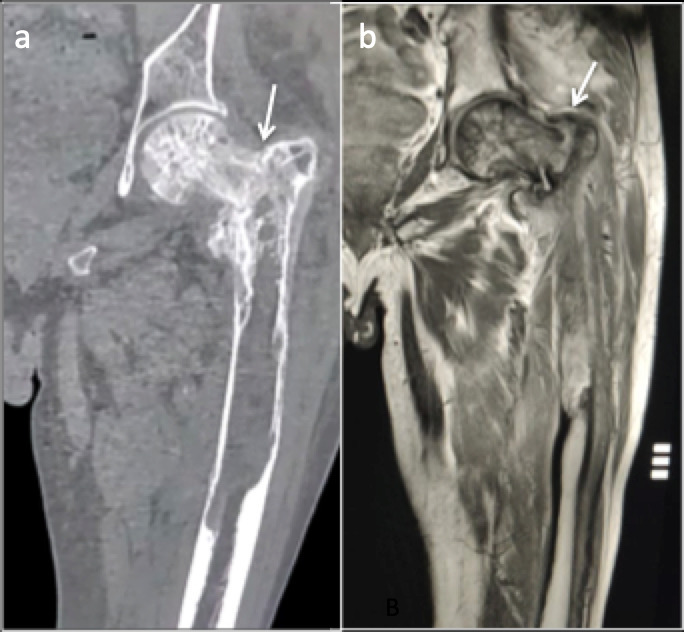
Paget’s disease of left proximal femur with slight bowing change and intertrochanteric fracture (arrows). (a) Coronal CT reformat and (b) Coronal MRI T1 imaging.
